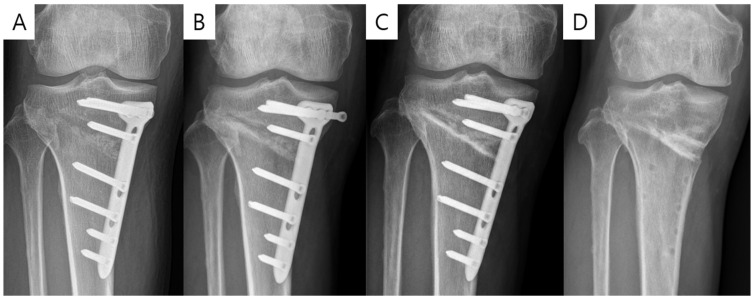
Radiography of screw loosening in a patient with conventional anatomic plate. (A) Immediate postoperative radiograph displaying D-hole violation. (B) Three-month postoperative radiograph showing proximal screw loosening and radiolucency around D-hole screw. (C) Radiograph following proximal screw reinsertion. (D) One-year postoperative radiograph.
